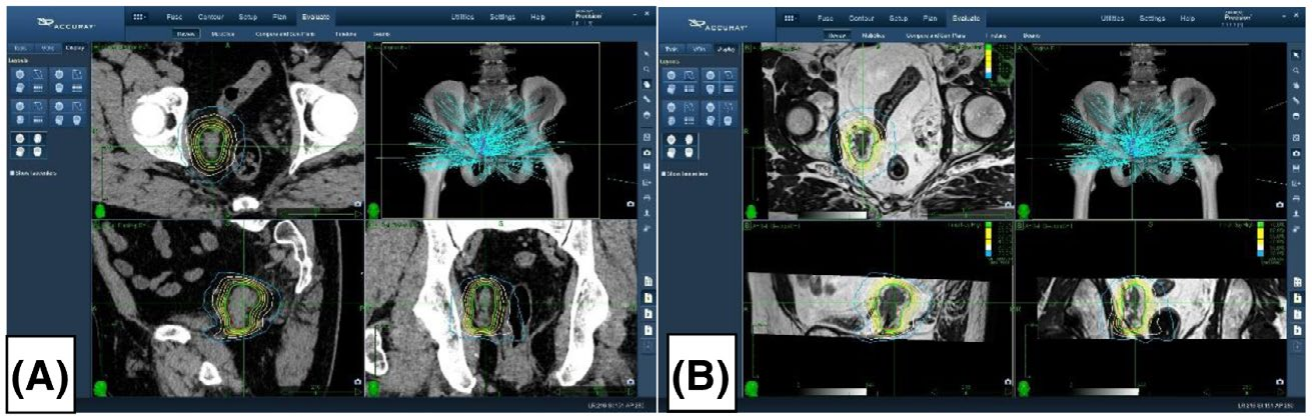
Treatment plan based on the planning CT (A), PET/CT and MRI (B) dataset
